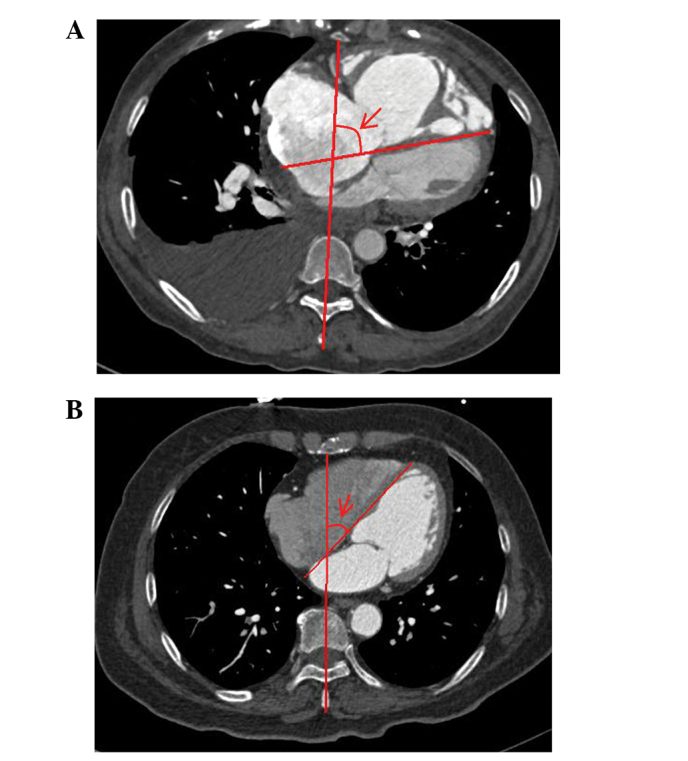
Septal angle measured on transverse images of computed tomographic pulmonary angiography (CTPA) in (A) a patient with CTEPH and (B) a normal patient. CTEPH, chronic thromboembolic pulmonary hypertension.
